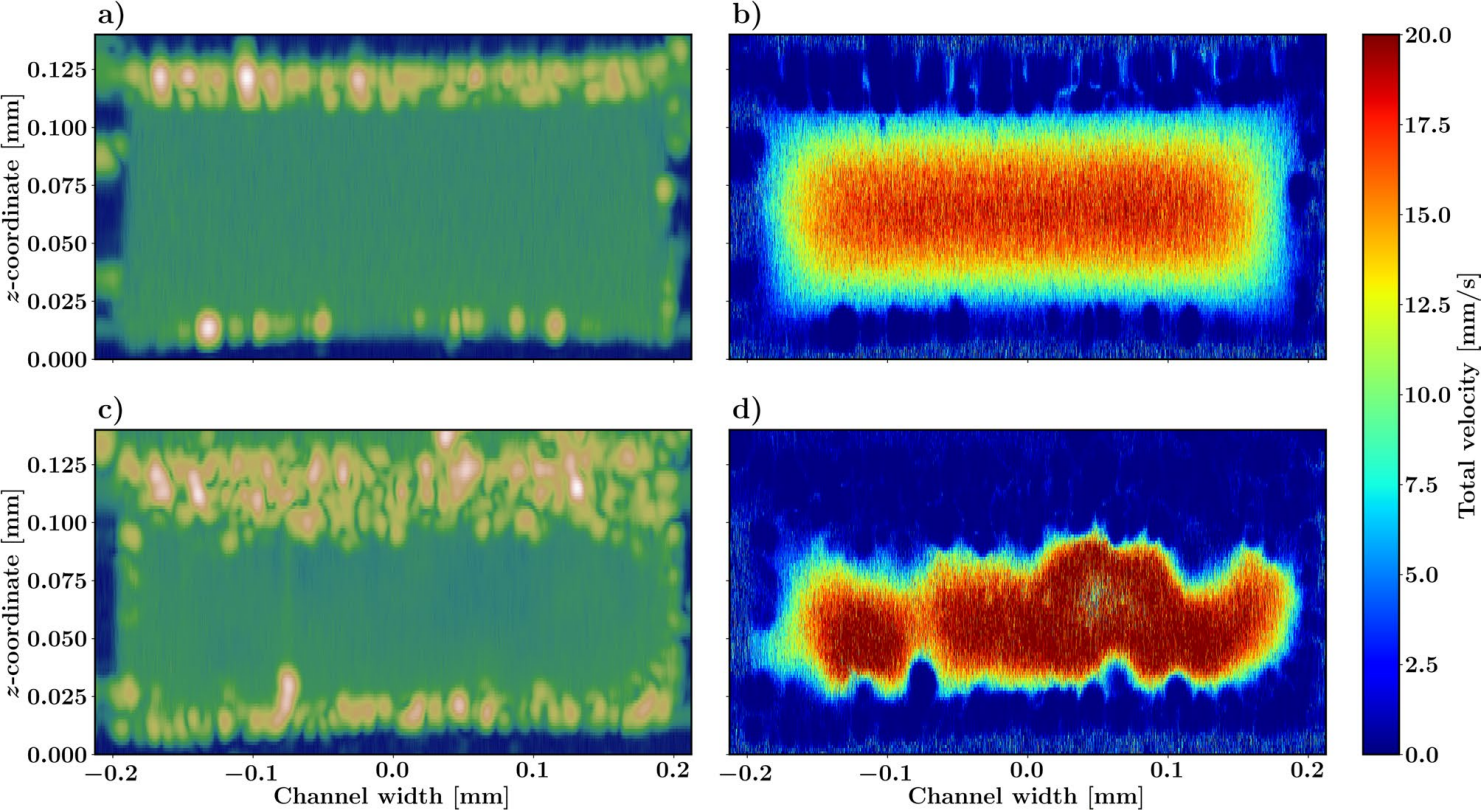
Cross-sectional B-scan intensity in dB scale perpendicular through the flow channel for the channel (a) with no pores and (c) with the presence of epithelial cells protruding from the culture well into the channel through the porous membrane. (b, d) Corresponding Doppler OCT flow measurements of the lumen.
